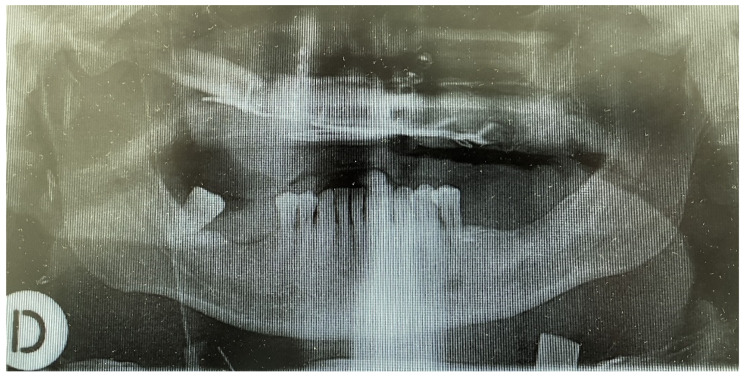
Post reconstruction panoral X-ray (The day after surgery).
